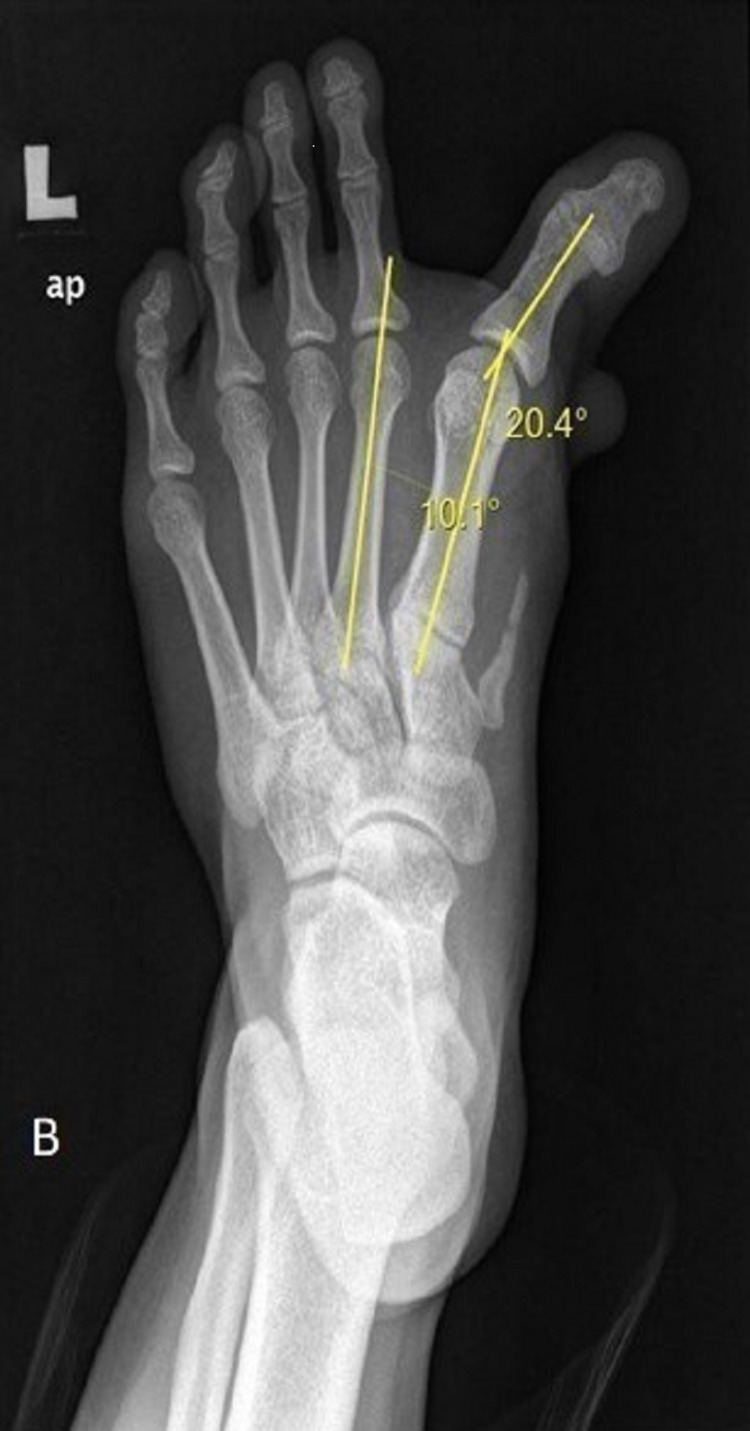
Preoperative X-ray of the left foot showing medial deviation of proximal phalanx of the great toe at the metatarsophalangeal (MTP) joint with an accessory bone over the medial cuneiform.
